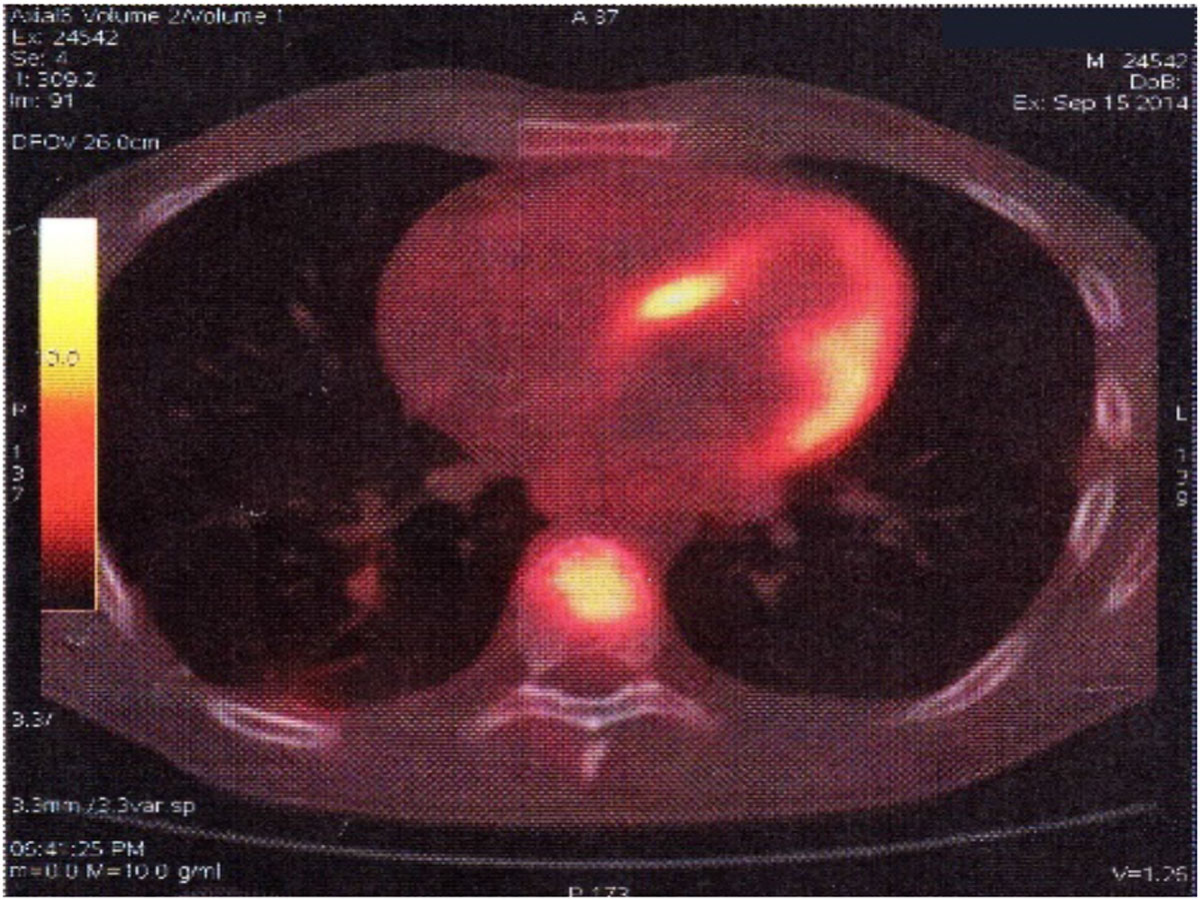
18F PET-CT scan. Lytic lesionin thoracic vertebrae body.
